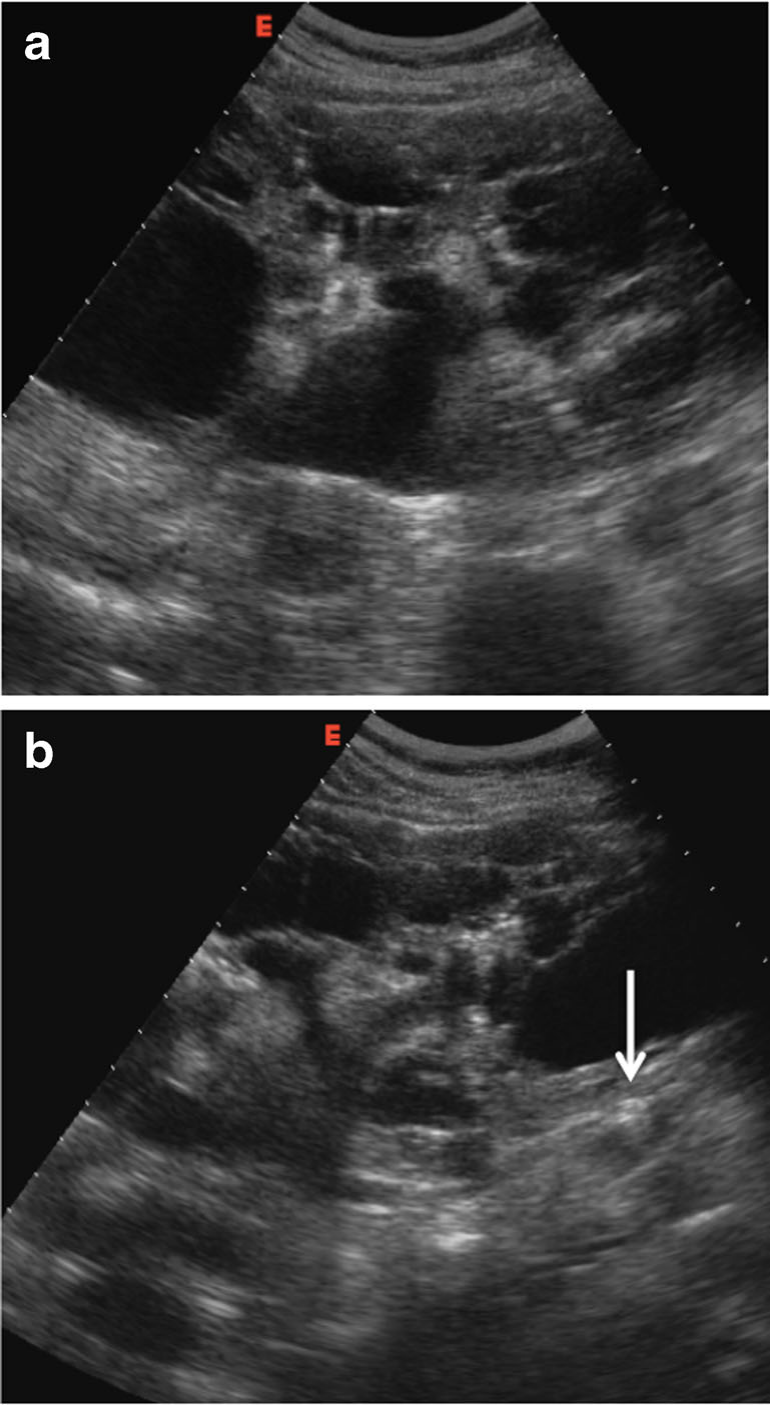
Patient with known congenital polycystic kidney disease and mild renal impairment with flank pain and acute renal insufficiency. a Right kidney with multiple cysts and mild dilatation of the pelvis; b minimal dilatation of the ureter due to a small stone (*arrow*) at the upper third of the ureterAdditional sonographic features that can help in the diagnosis.
